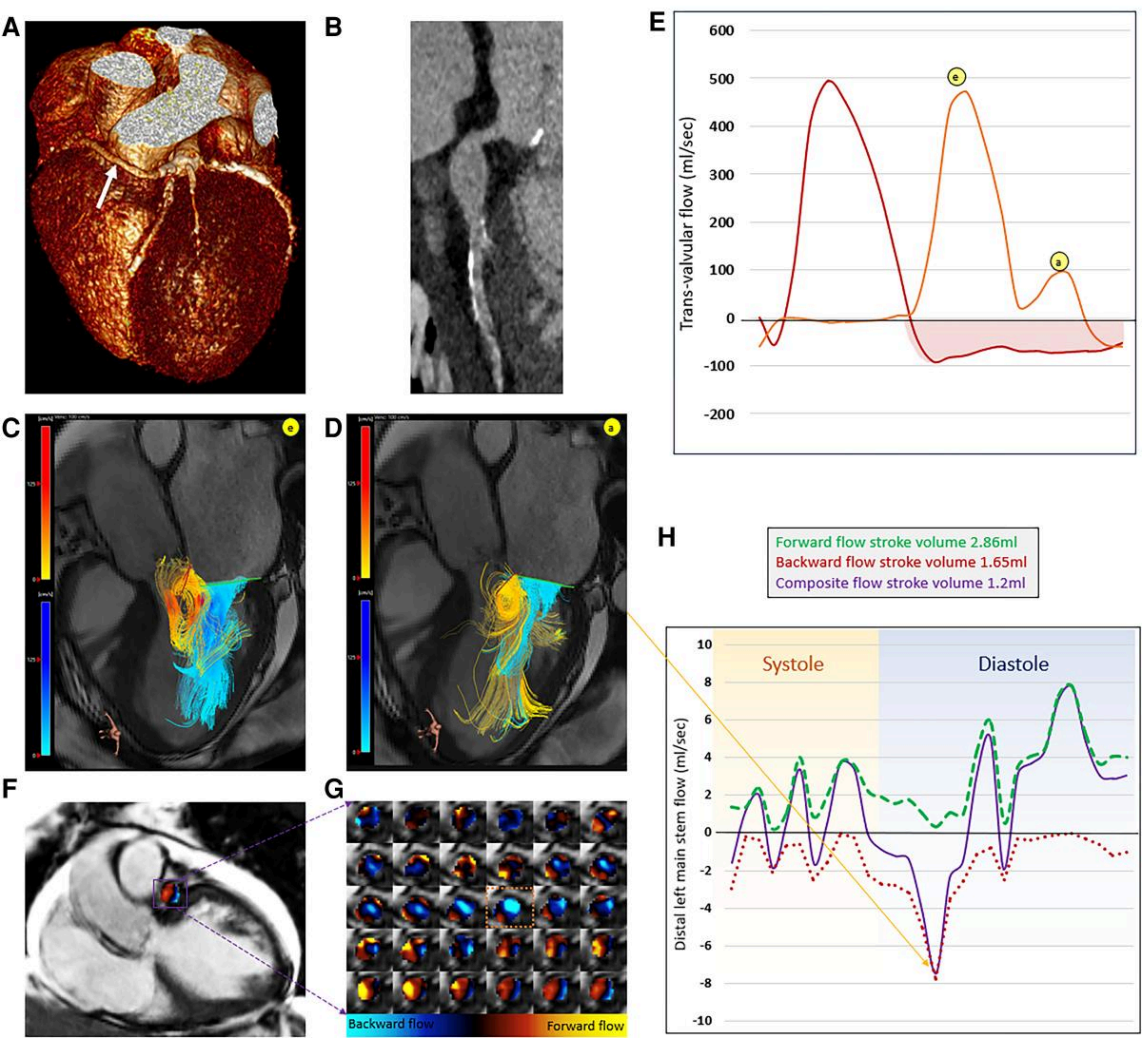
Four-dimensional flow imaging (4D flow) cardiovascular magnetic resonance imaging of aortic regurgitation and coronary artery. (*A*) Three-dimensional reconstruction of the heart and coronary arteries using cardiac computed tomography demonstrating that the right coronary artery originates from the left main stem (LMS) (white arrow). (*B*) Multi-planar reconstruction of the LMS and proximal left anterior descending artery demonstrating LMS dilatation. (*C*) Three-dimensional blood flow streamlines demonstrating competing aortic regurgitation (red-yellow scale) vs. mitral inflow blood flow (blue scale) during early filling. (*D*) Three-dimensional blood flow streamlines demonstrating competing aortic regurgitation (yellow) vs. mitral inflow blood flow (blue) during late filling. The aortic regurgitation interferes with the opening of the anterior mitral valve leaflet and restricts the filling. (*E*) Transvalvular flow curves (mitral, orange flow curve; aortic, red flow curve) computed using valve tracking procedures demonstrate significant holo-diastolic aortic regurgitation. (*F*) A coronal view cine where dilated left main step was identified and corresponding 4D flow data were then superimposed. (*G*) The LMS flow for the complete cardiac cycle (beginning with systole similar to flow curves in panel *E*) demonstrates backward flow during diastole as blue. (*H*) Coronary flow quantification flow curves demonstrate bi-phasic flow reversal during diastole—which is abnormal and mainly due to negative pressure in the aortic sinus, resulting from aortic regurgitation.
